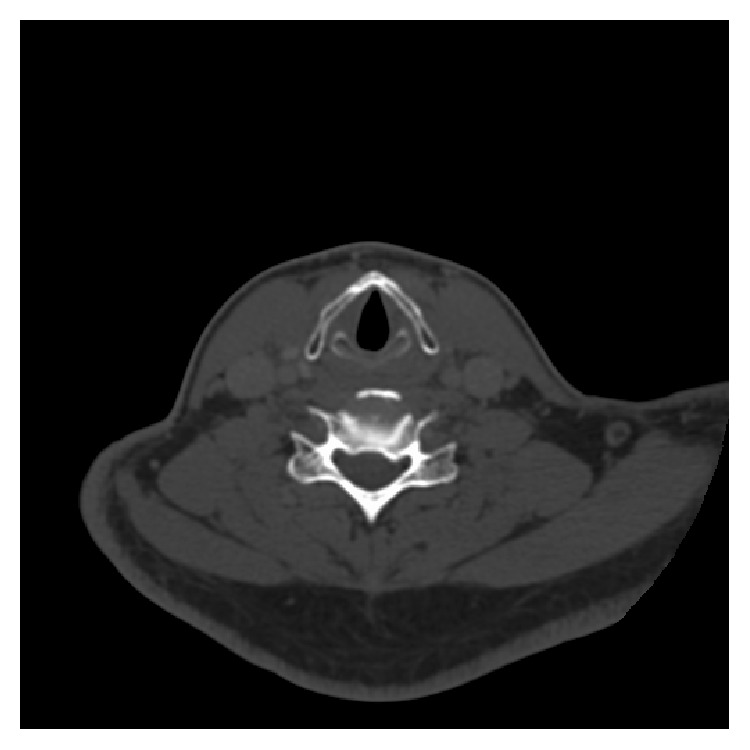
Axial computed tomography image of radiation recurrent glottic cancer shows no evidence of invasive disease into the thyroid cartilage prior to initial salvage surgery.
